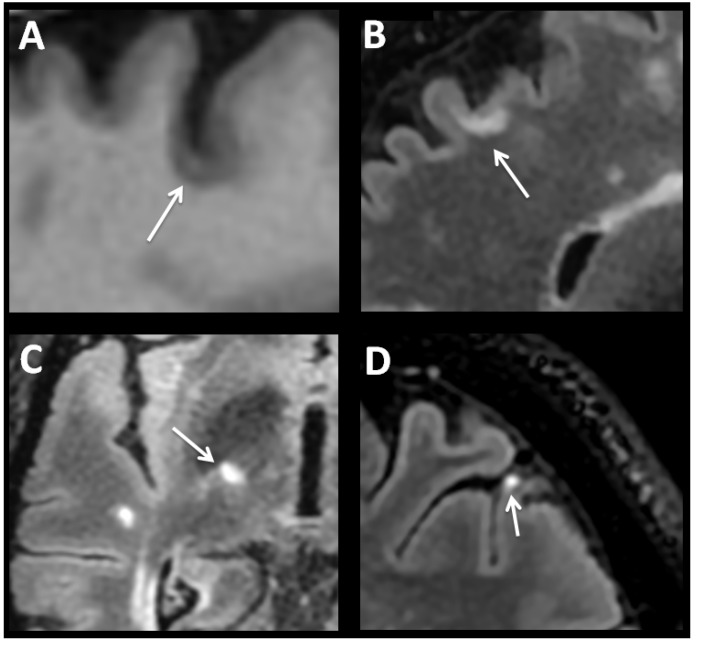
(A) Three-dimension sagittal T1-weighted. Hypointense cortical lesion (white arrow). (B) Three-dimension sagittal T2-Fluid Attenuated Inversion Recovery (FLAIR). Hyperintense leukocortical lesion (white arrow). (C) Axial FLAIR. Subcortical temporal demyelinating plaque and perithalamic internal capsule lesion (white arrow). (D) Post-contrast 3D sagittal FLAIR. Focal area of leptomeningeal enhancement (white arrow).
